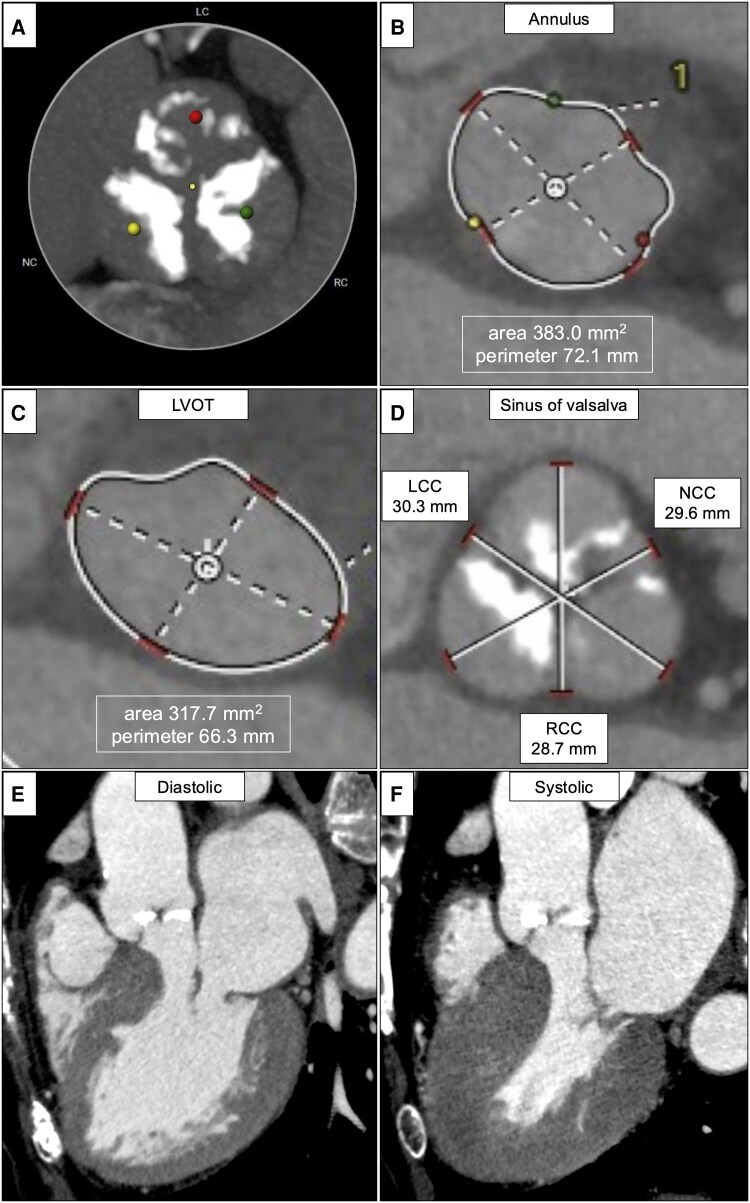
Preprocedural computed tomography (CT) findings of the periaortic anatomy and left ventricle. (*A*) Tricuspid aortic valve with severe calcification. (*B–D*) CT images showing anatomical structures surrounding the aortic valve and relevant measurements. (*E*, *F*) Diastolic and systolic long-axis CT views showing a bulging basal interventricular septum. The anterior mitral leaflet does not exhibit systolic anterior motion and asymmetric septal hypertrophy is not evident. Abbreviations: LCC, left coronary cusp; LVOT, left ventricular outflow tract; NCC, noncoronary cusp; RCC, right coronary cusp.
